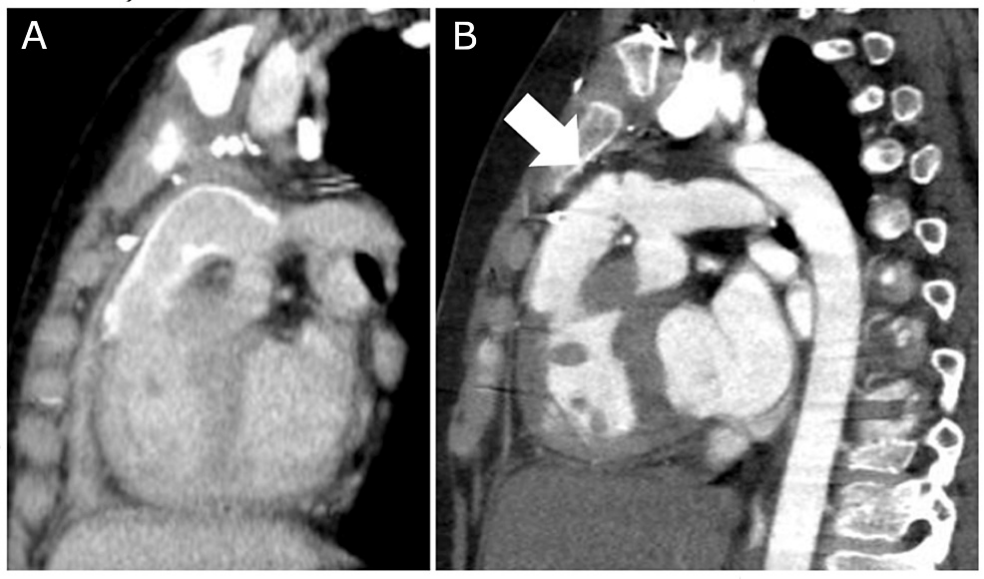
Sagittal section of computed tomography: (A) 10 days and (B) five years after re-operation. An additional tract is curved tightly, bent at the valve just above the coronary artery (white arrow) after five years.
